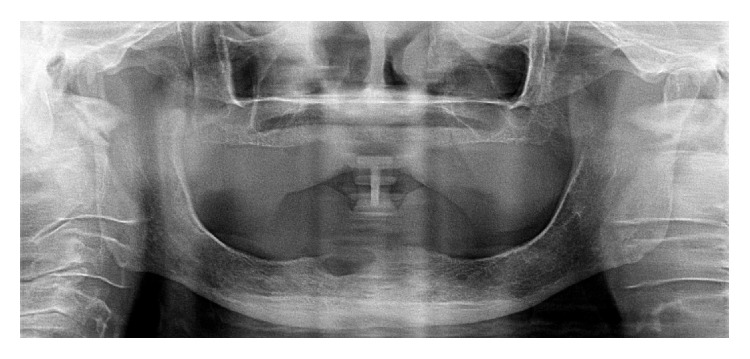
Panoramic radiography showing an unilocular radiolucent lesion in anterior mandible.
